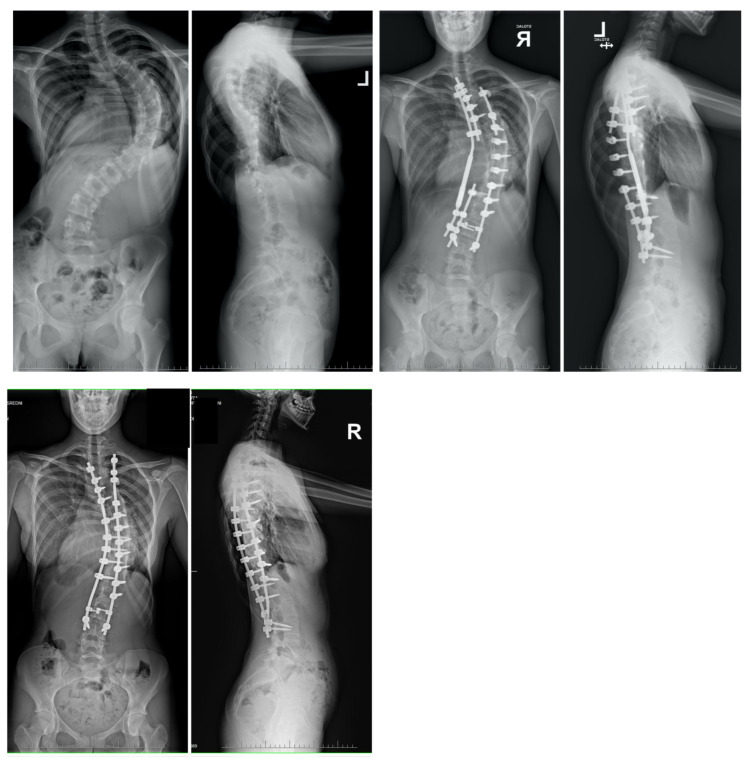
During the observation period, pre- and post-operative radiographs were utilized to monitor the treatment progress of a 16-year-old female diagnosed with severe adolescent idiopathic scoliosis. Staged surgery was conducted, involving a temporary internal distraction device with MCGR for initial intervention, followed by the implementation of double Co-chr 6.0 rods for final correction of the spinal curvature.
